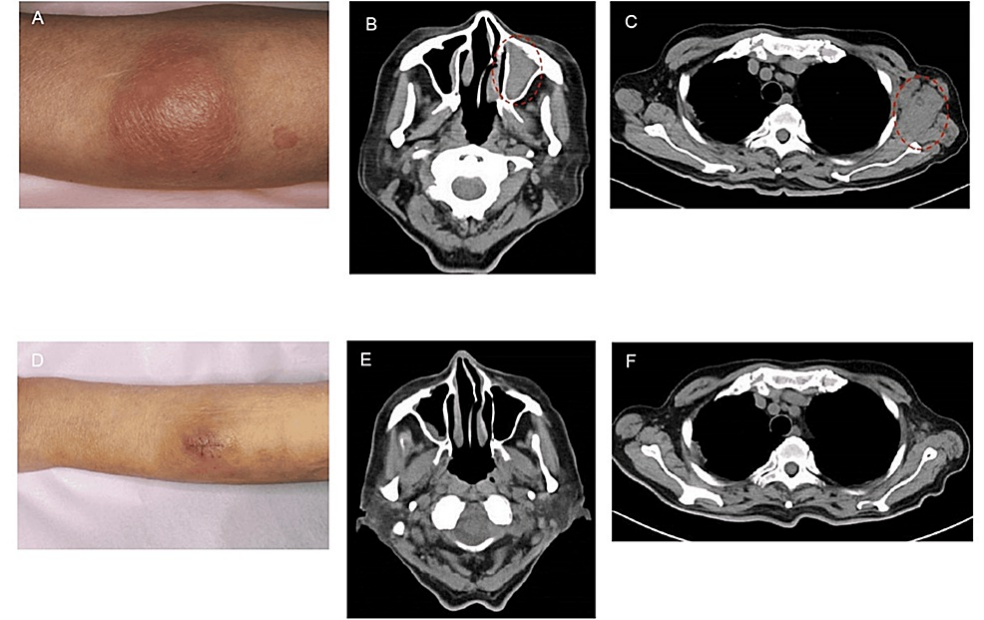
Subcutaneous tissue of the left antebrachium, soft shadow in the left maxillary sinus on cervical computed tomography before and after venetoclax/azacitidine treatment. A subcutaneous tissue of the left antebrachium with a size of 60 × 30 mm (A), a soft shadow in the left maxillary sinus on cervical computed tomography (CT) (B), and a mass in the left axilla on thoracic CT (C) observed on admission. After the first venetoclax/azacitidine therapy cycle, a scar on the subcutaneous tissue with a size of 28 × 24 mm (D) was observed, and soft shadow (E) and mass in the left axilla (F) disappeared.
